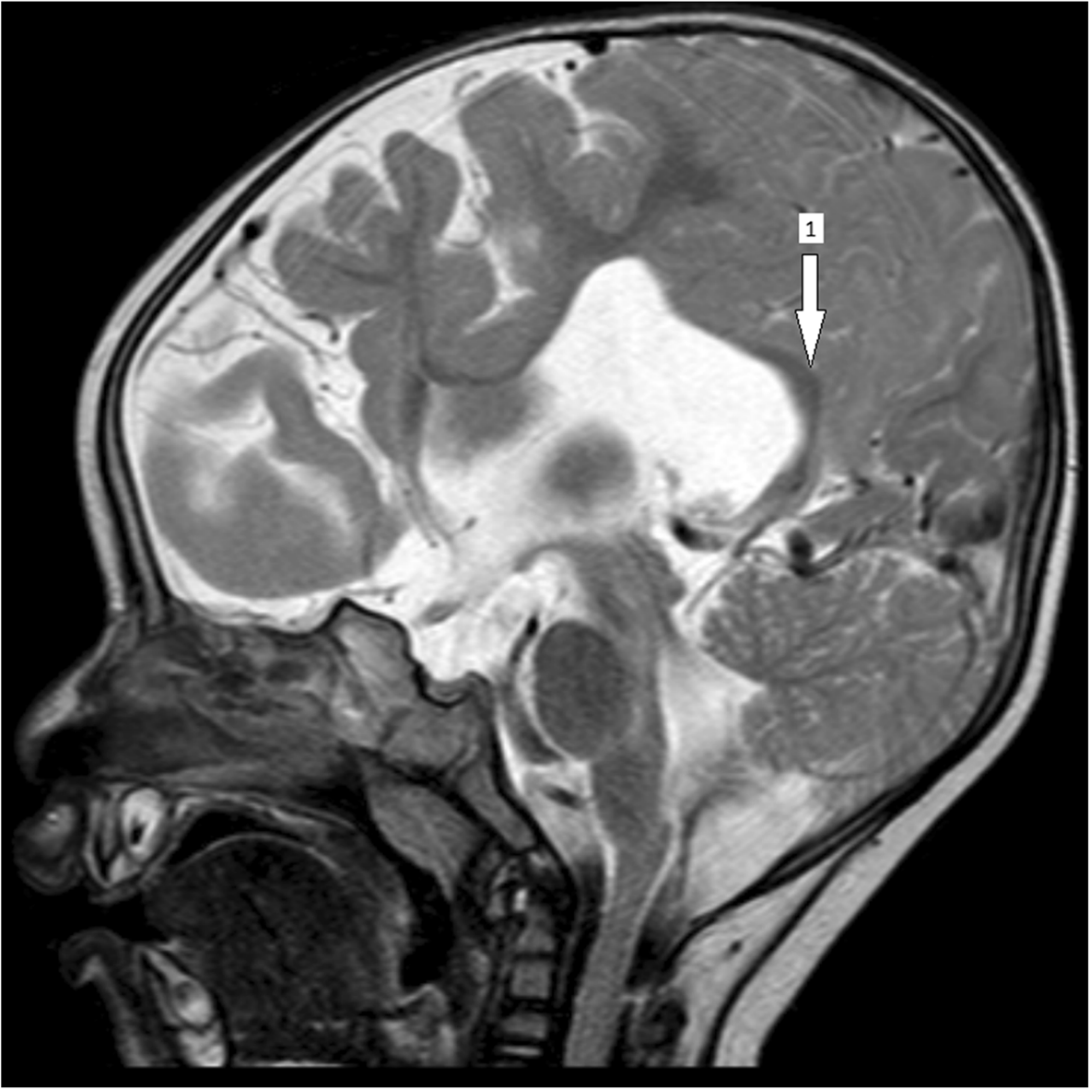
Brain magnetic resonance imaging (T2 weighted) displaying a partially formed corpus callosum (*arrow 1*)
